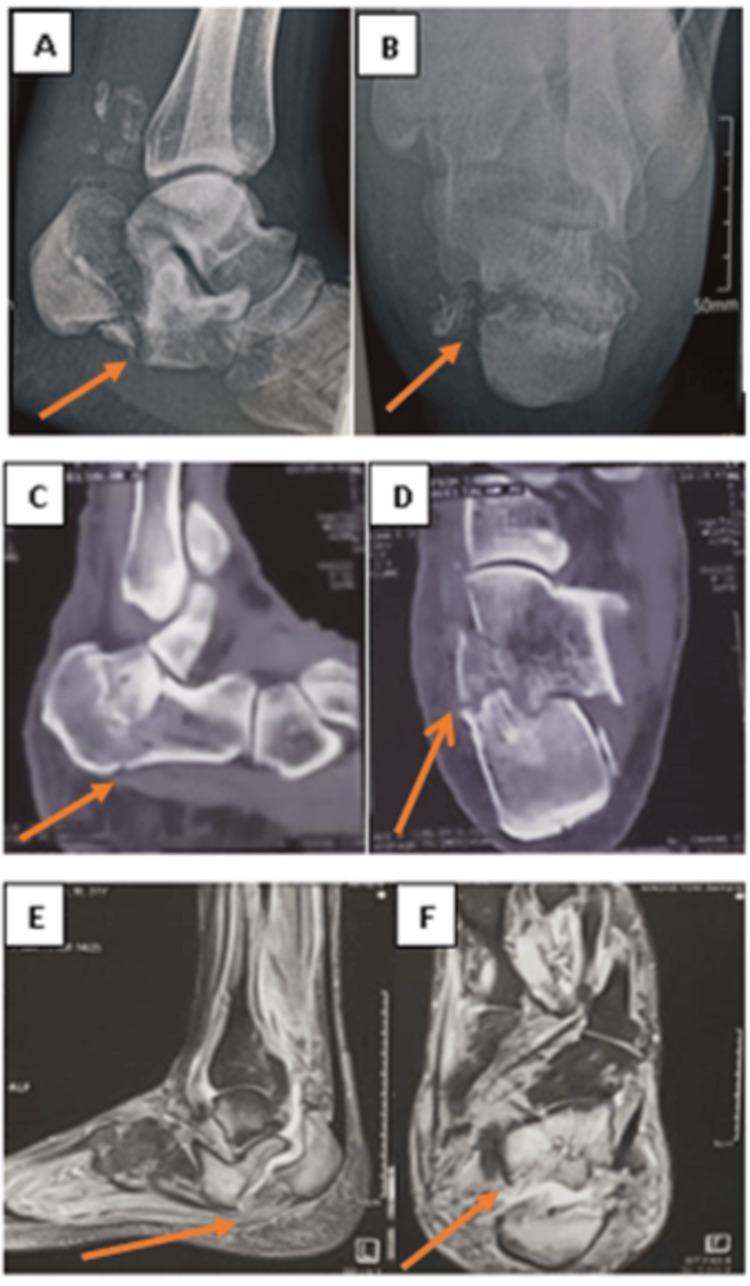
Preoperative images of the fracture included. Plain X-ray: (A) lateral view and (B) axial view. CT scan: (C) sagittal view and (D) semi coronal view. MR images: (E) sagittal view and (F) axial view
